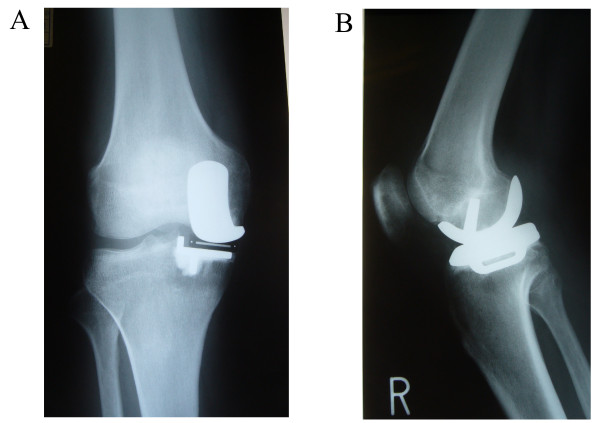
A: anteroposterior, B: lateral plain radiographs. No formal signs of loosening such as radolucent lines or osteolysis.
